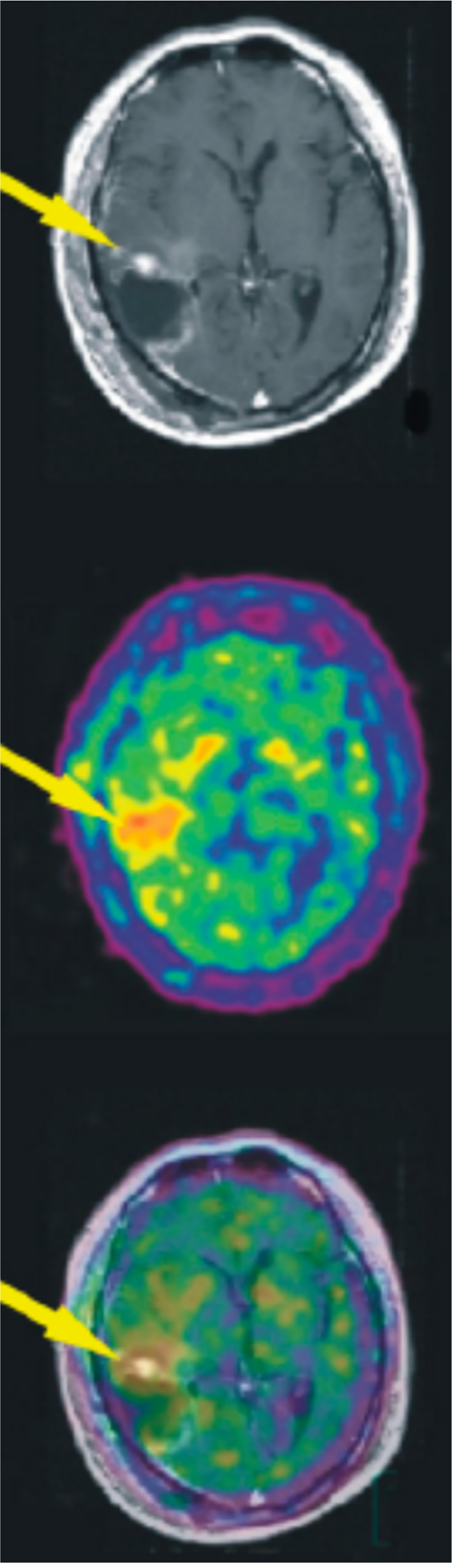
S*-[^11^C]*Methyl*-*l*-cysteine MRI, PET, and coregistered PET/MRI
